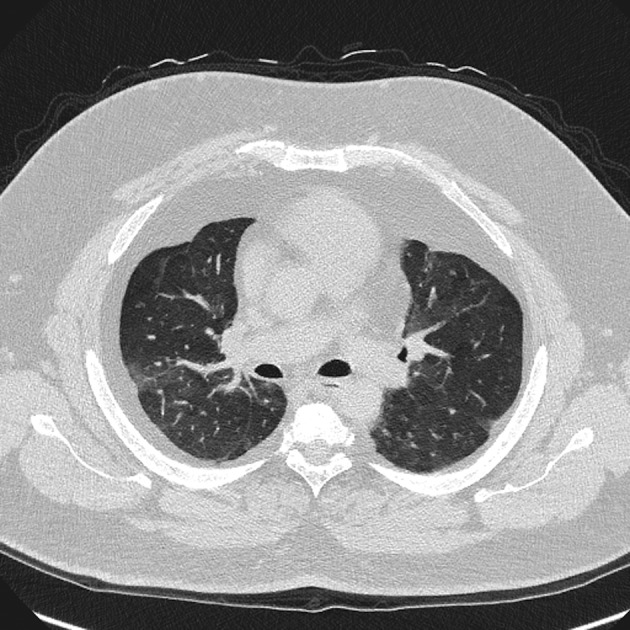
Computed tomography chest showing extensive mediastinal and pleural fat accumulation.
